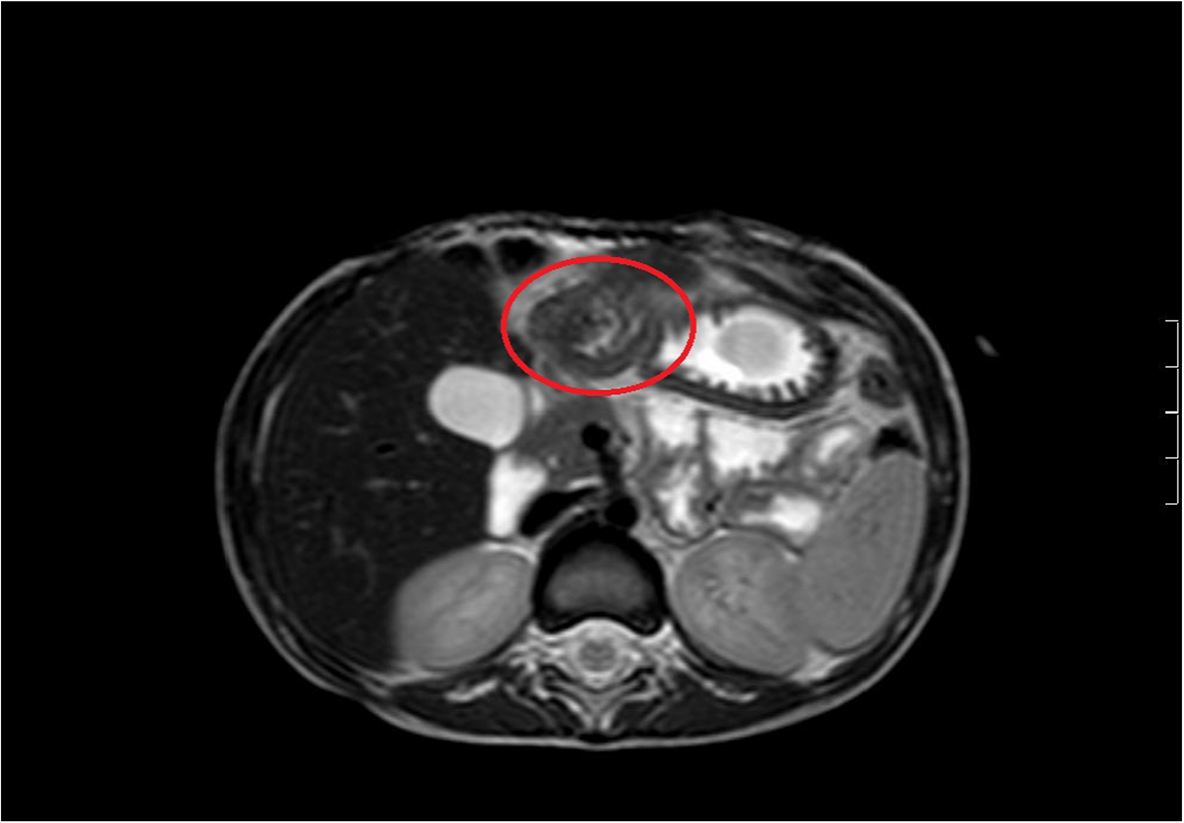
Magnetic resonance imaging of gastro-pyloric heterotopic pancreas (circle)
